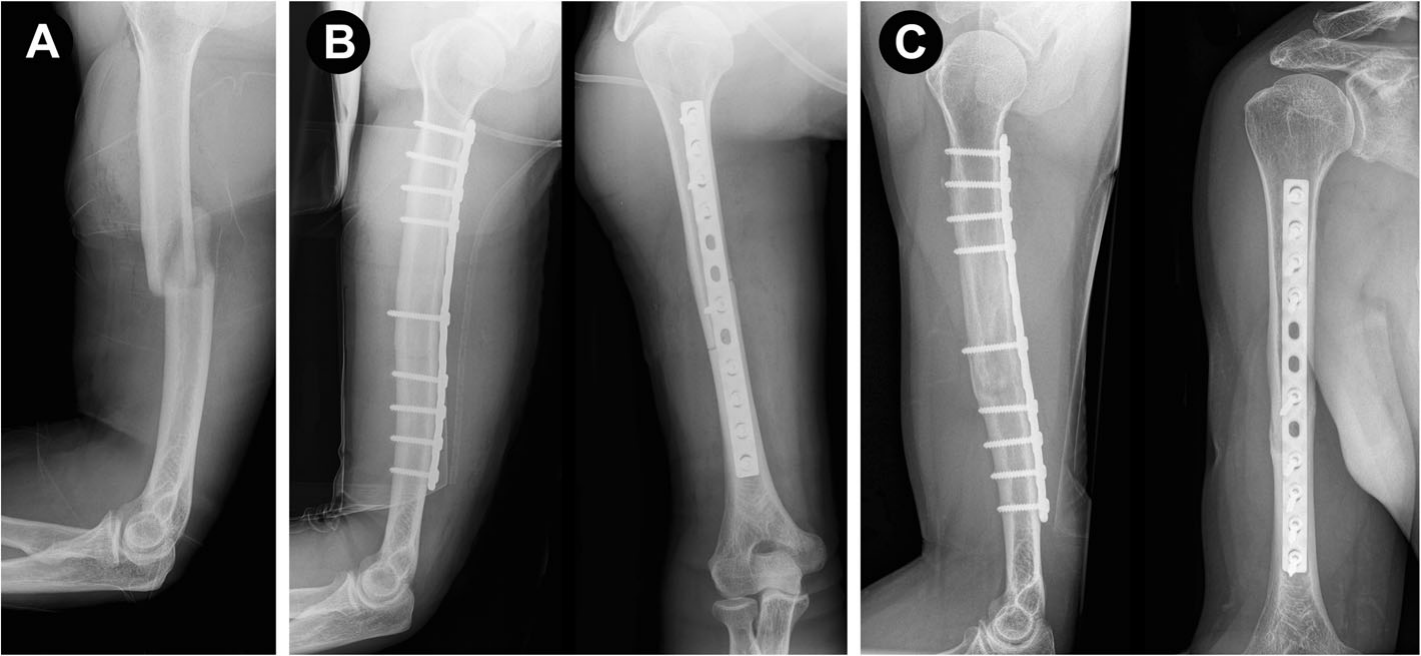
a–c. A 55-year-old patient with an Orthopaedic Trauma Association (OTA) type 12-A1 humeral fracture (a). Postoperative X-rays after open reduction and internal fixation (ORIF) using a limited contact dynamic compression plate (LCDCP) (b). Final anteroposterior and lateral views showing secondary fracture healing with callus formation as a result of relative stability of the construct (c)
